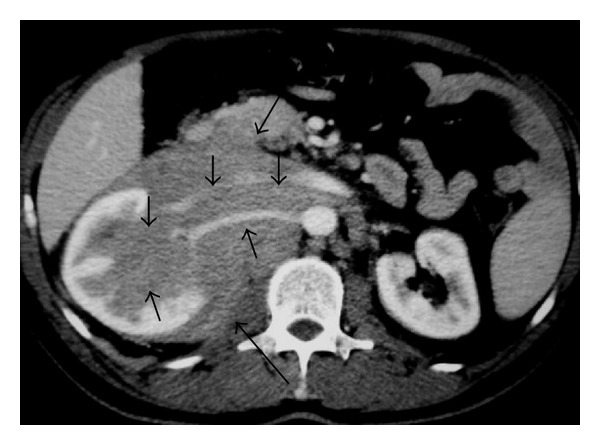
NHL. Axial contrast-enhanced CT reveals soft-tissue mass involving the right kidney (long arrows) with lymphomatous infiltration of the renal sinus and encasement of vessels (short arrows).
